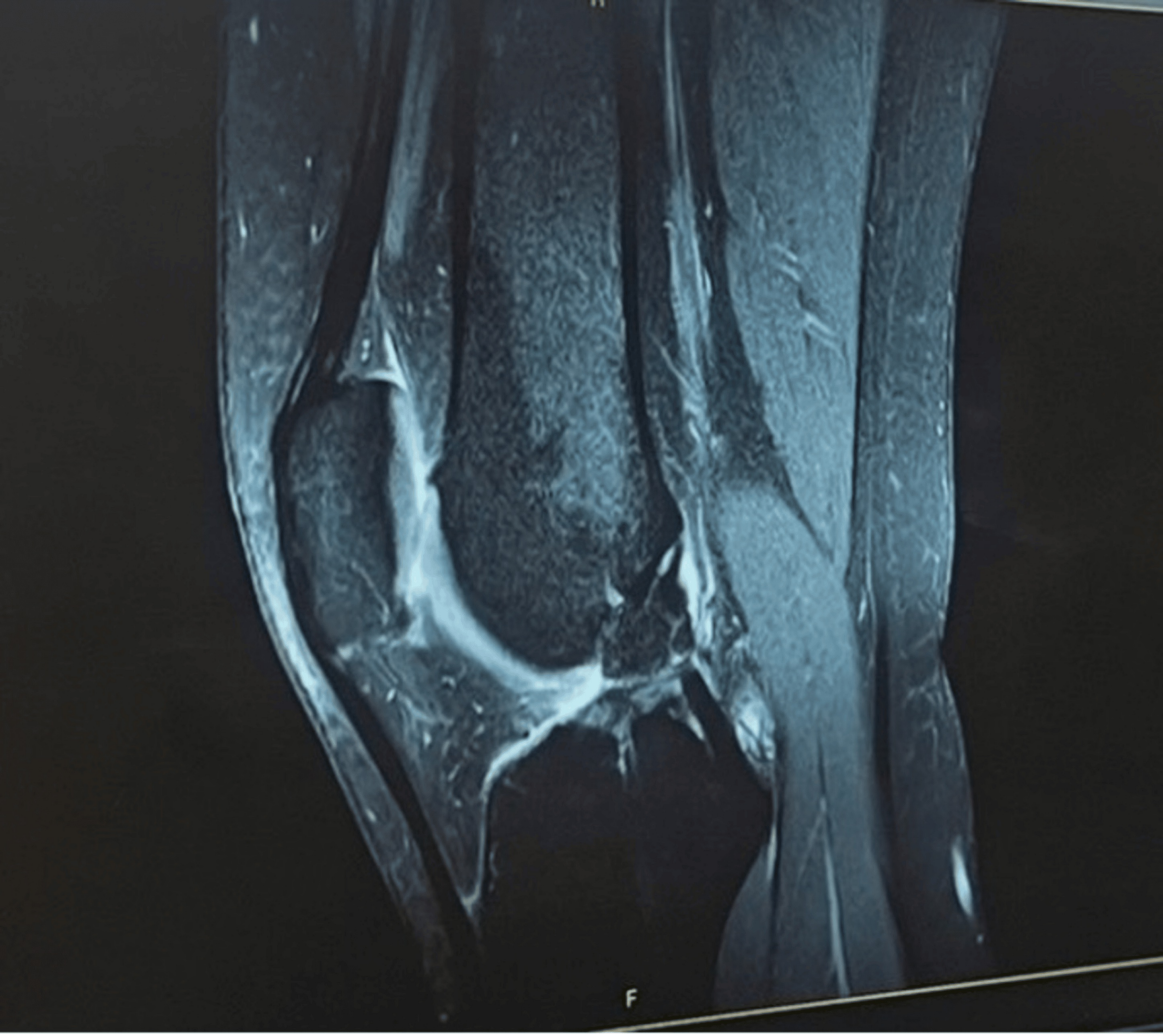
Coronal MRI of the right knee demonstrating lateral compartment findings MRI: magnetic resonance imaging
